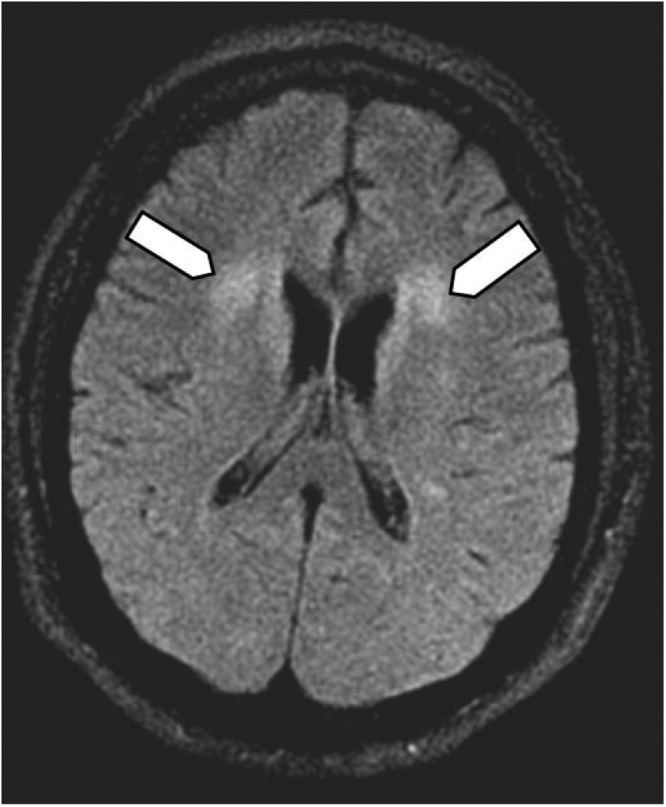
Initial non-enhanced MRI of the brain. Axial FLAIR sequence demonstrates bilaterally prominent periventricular white matter signal hyperintensities (white arrows).
